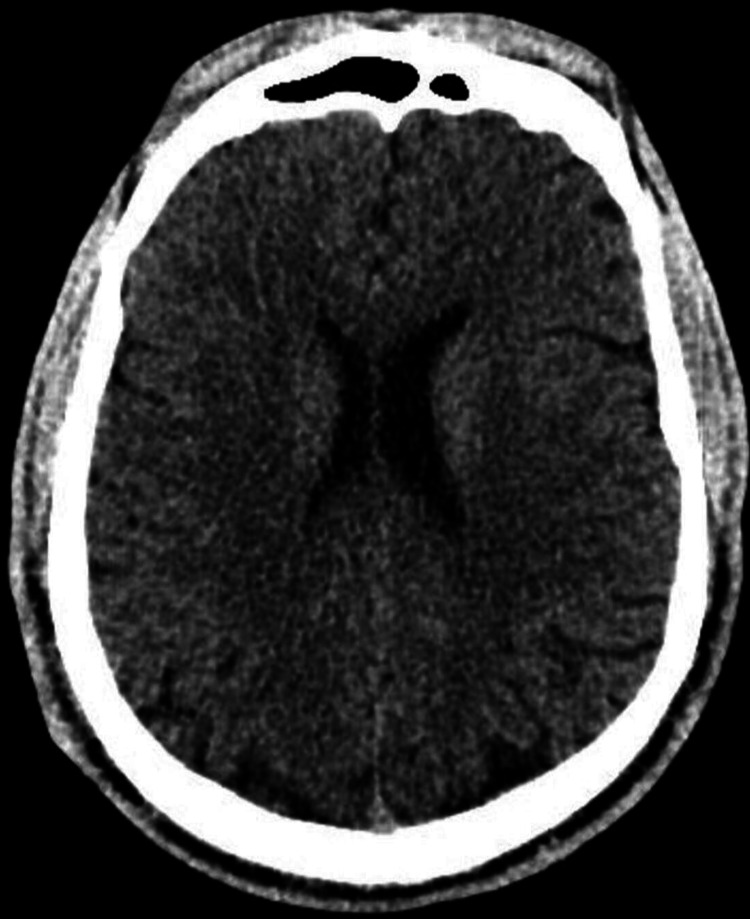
Head CT scan of the first patient (FR) This non-contrast axial head CT shows a normal study at the level of the frontal horns of the lateral ventricles. The frontal sinuses are well-aerated anteriorly, and the cerebral hemispheres appear symmetric with preserved gray-white matter differentiation, indicating no evidence of acute ischemia or cerebral edema. The lateral ventricles are normal in size and shape, with no signs of hydrocephalus or midline shift. The septum pellucidum is midline and intact. There is no evidence of intracranial hemorrhage, mass effect, or extra-axial collections. CT: computed tomography
